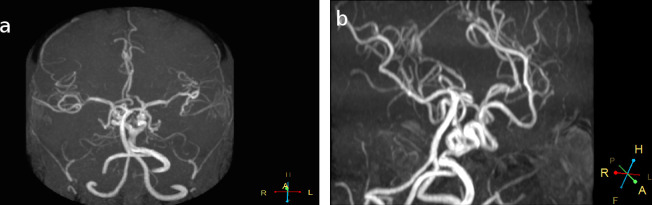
Coronal & sagital reformatted MIP MRI images demonstrationg the extracranial fusion of right and left ICA into a single vessel. It divides into very tortuous intracranial ICA’s. ICA, internal carotid artery; MIP, maximum intensityprojection.
